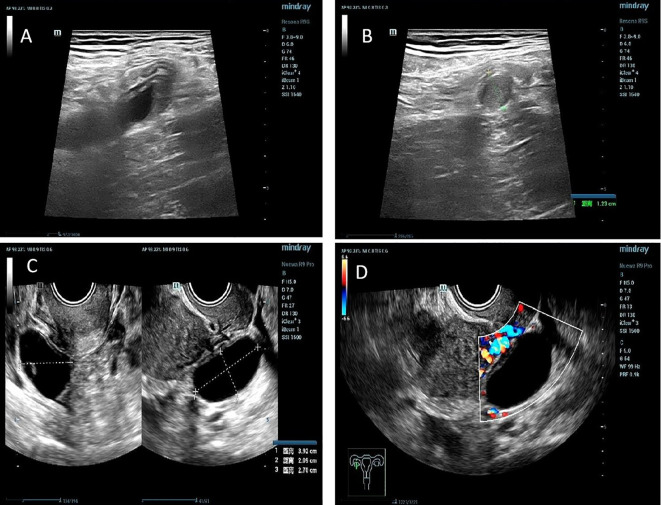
Ultrasound examination of the appendix and parovarian cyst. (A, B) Ultrasound revealed significant thickening of the appendix, with a maximum diameter of 1.23 cm and poor anechoic transmission in the lumen. (C, D) Anechoic area was detected in the right adnexal region, measuring approximately 3.92 cm × 2.70 cm × 2.09 cm.
